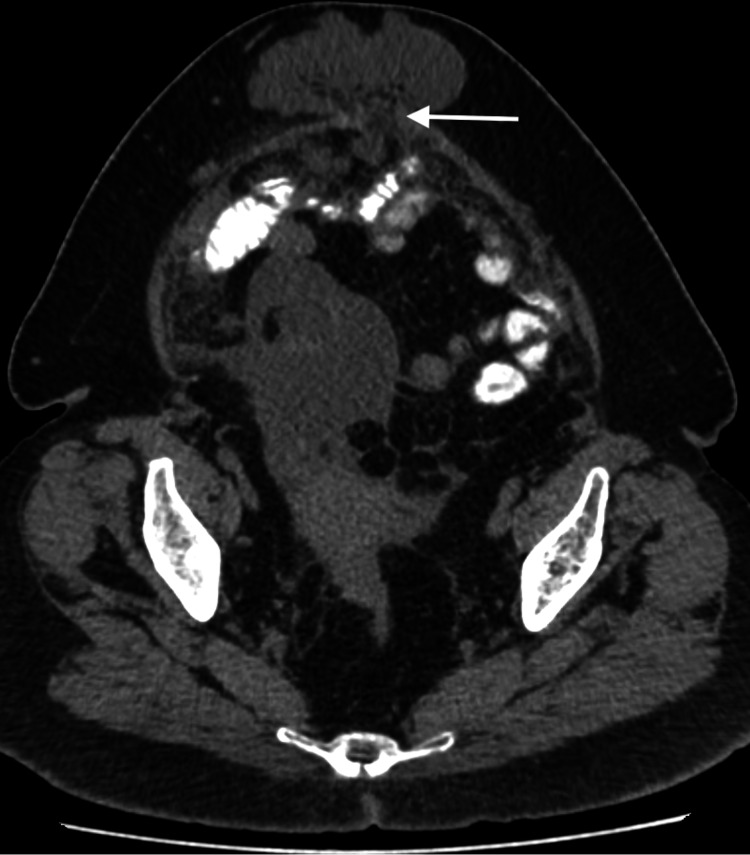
Axial CT imaging of the lower abdomen with oral contrast. Axial view of the lower abdomen demonstrates extension of the mass from the right lower quadrant to the anterior abdomen. The mass is seen herniating through a defect in the anterior abdominal wall (arrow). Fat stranding is also seen at the neck of the hernial sac.
